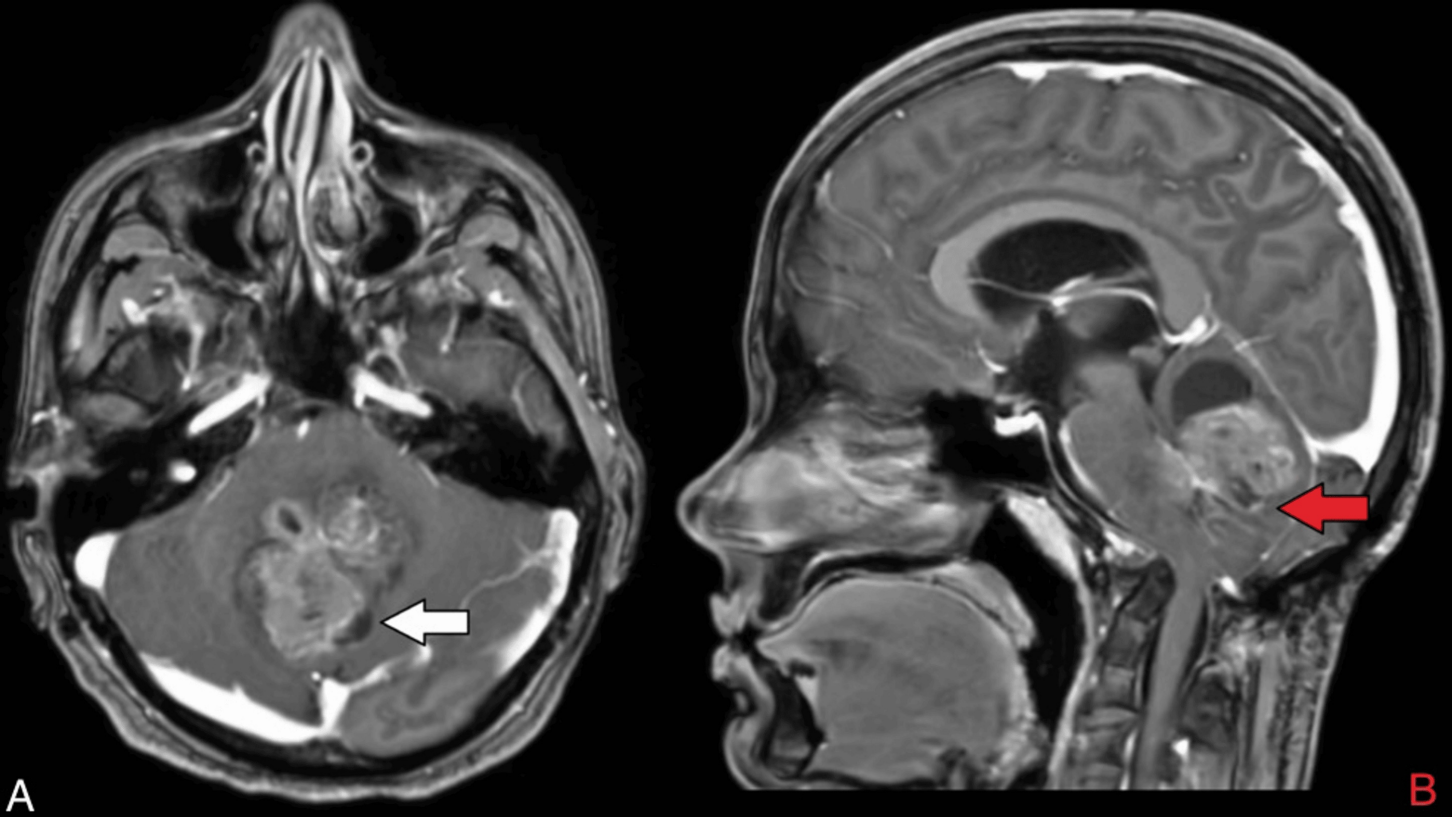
MRI brain T1 contrast axial and sagittal images A) The white arrow shows a heterogeneous signal intensity solid lesion with few pseudo-cystic areas in the posterior fossa seen involving the vermis and roof of the fourth ventricle, bilateral cerebellar peduncles and causing a mass effect in the form of perilesional oedema and anterior displacement of the brain stem. B) The red arrow shows compressing the vermis, pons, and medulla, leading to upstream dilatation of the third and bilateral lateral ventricles. MRI: Magnetic resonance imaging
